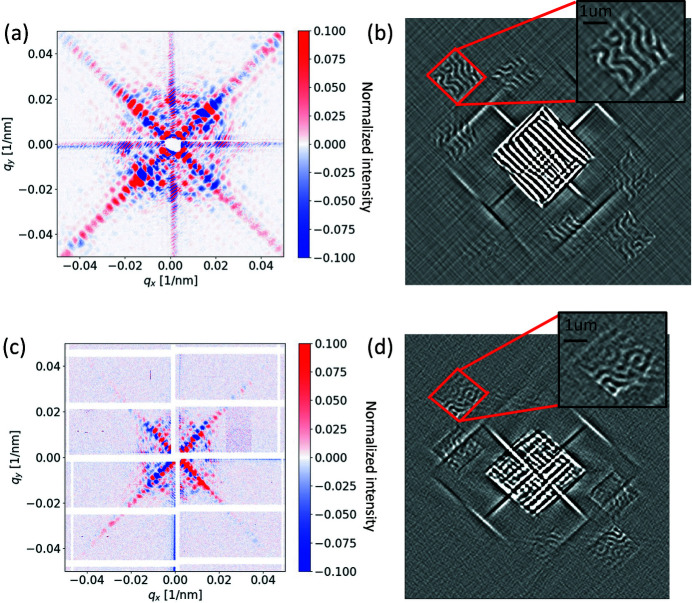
Megahertz-rate magnetic X-ray holographic imaging. Magnetic hologram of a CoFeB thin film multilayer recorded at the Fe *L*
